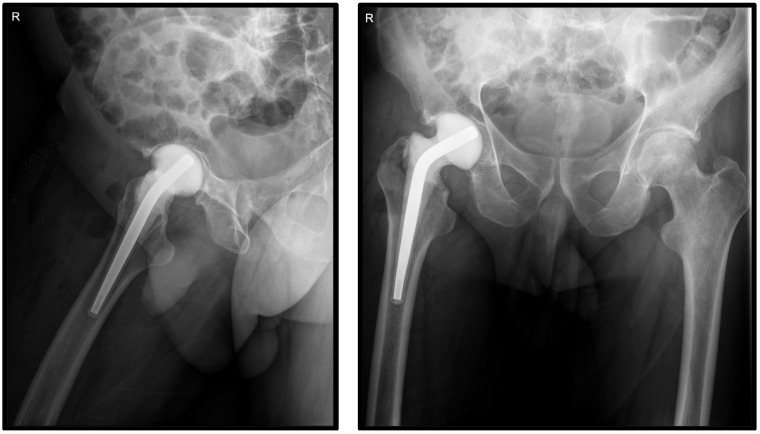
Postoperative X-ray assessment showing the antibiotic-loaded spacer.
